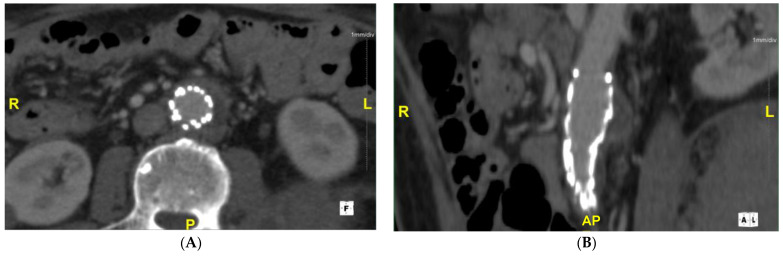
CT scan (A) axial view and (B) coronal view at 6 weeks after endovascular repair, demonstrating a stable sac without any noticeable evidence of mycotic aneurysm progression or endoleak. R = right; L = Left; P = Posterior, AP = Anterior Posterior.
